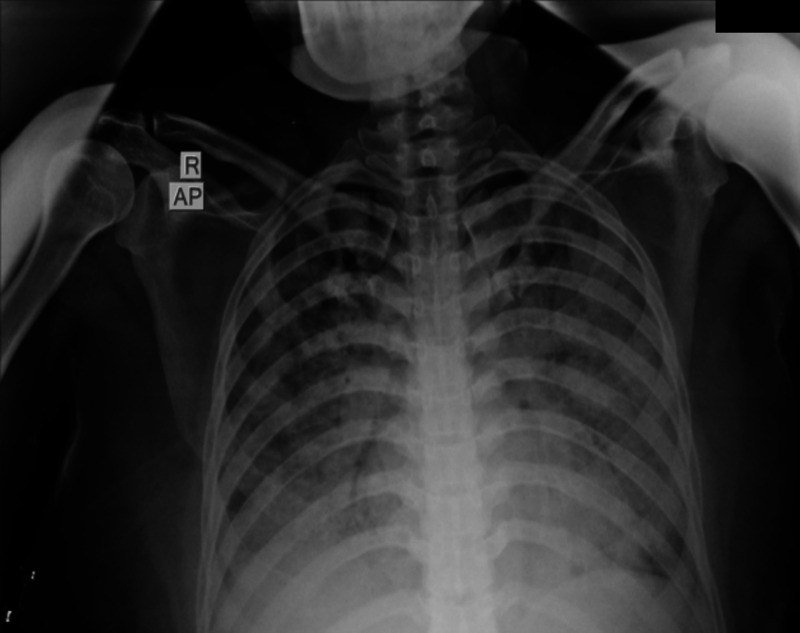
Chest radiograph (anterior–posterior view) of Case 1 showing bilaterally symmetrical diffuse alveolar opacities.
